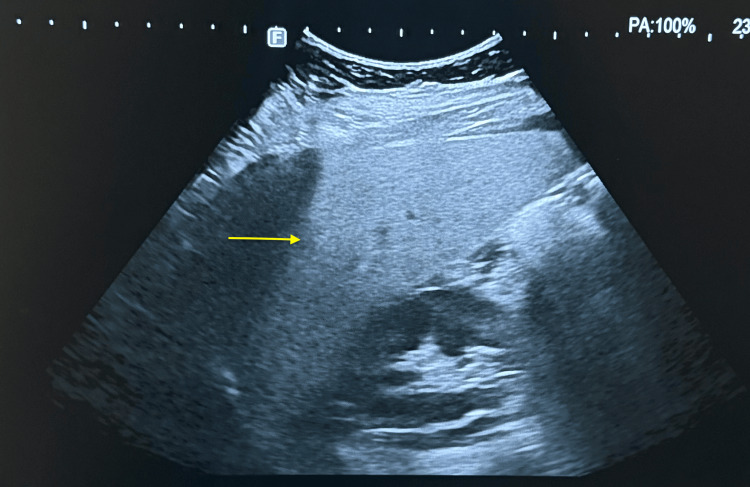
Ultrasound findings of hepatic steatosis Ultrasound image showing increased hepatic echogenicity compared with the right kidney, consistent with steatosis (yellow arrow indicating the liver).
